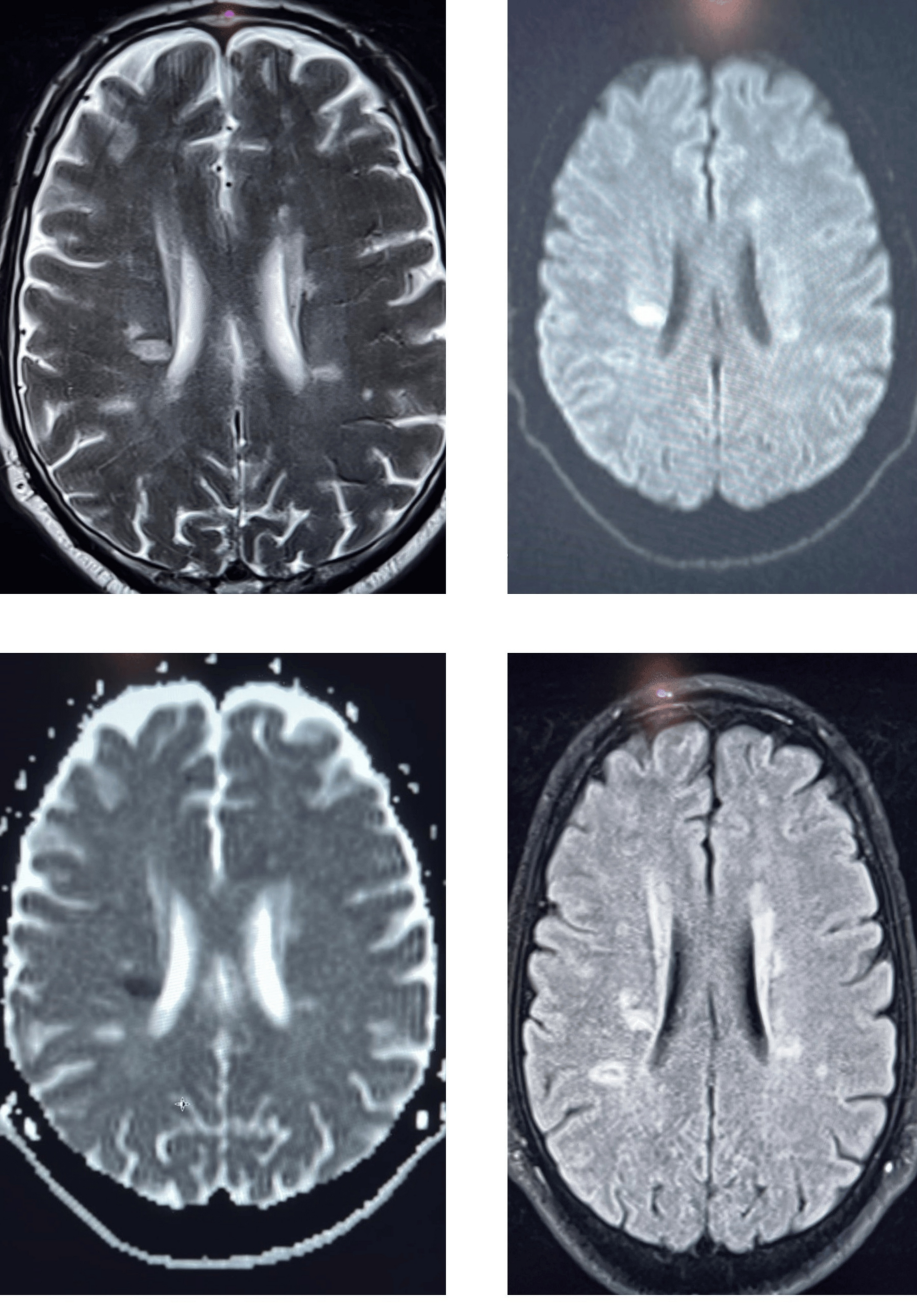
MRI findings in multiple sclerosis
